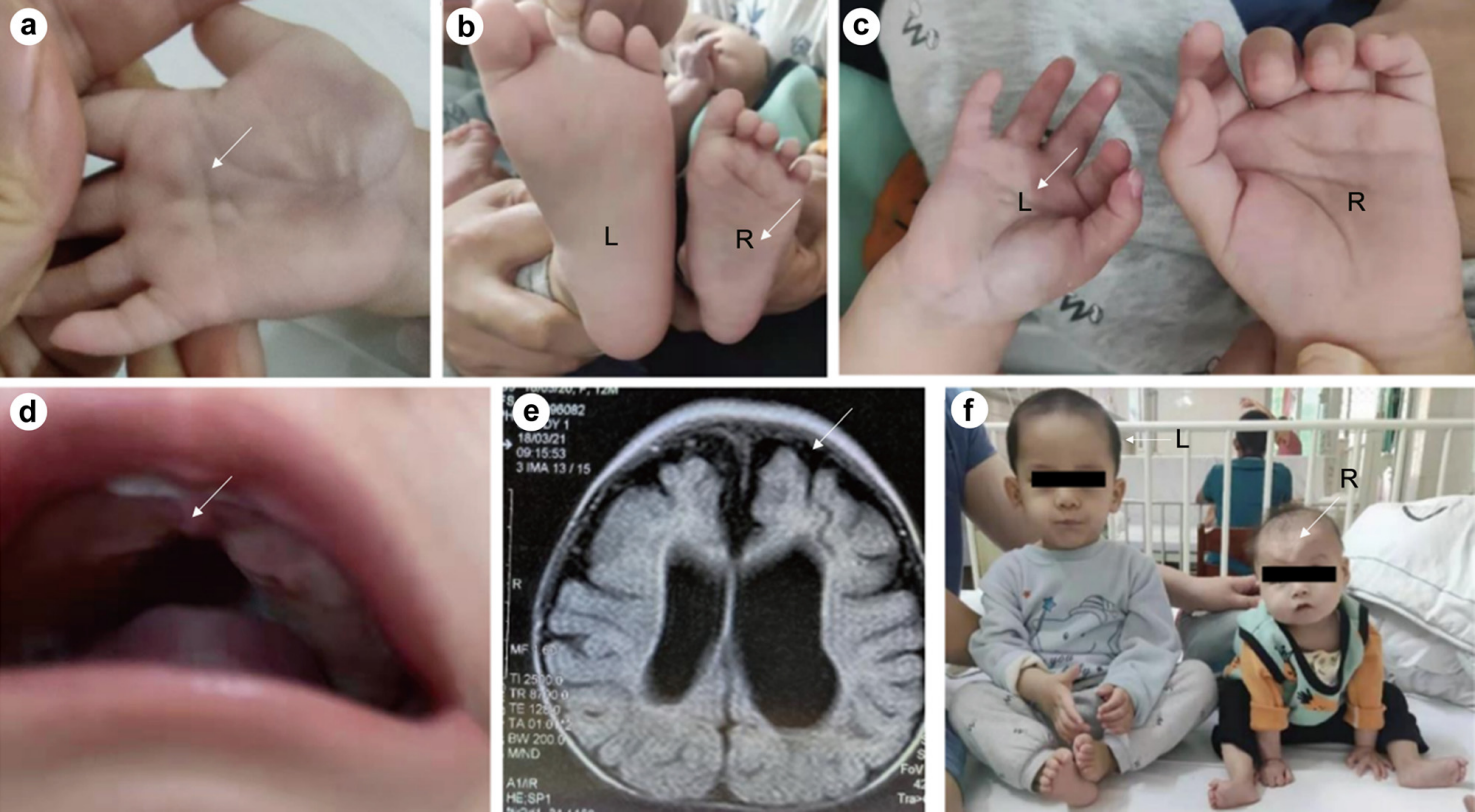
Physical features and MRI of the brain of the girl. (a) Simian crease was found in the right palm; (b) comparison of the foot size between the patient (R) and healthy counterpart (L); (c) comparison of the palm size between the patient (L) and healthy counterpart (R); (d) I° cleft palate; (e) MRI findings of hydrocephalus; (f) comparison of physical characteristics between the patient (R) and healthy counterpart (L).
